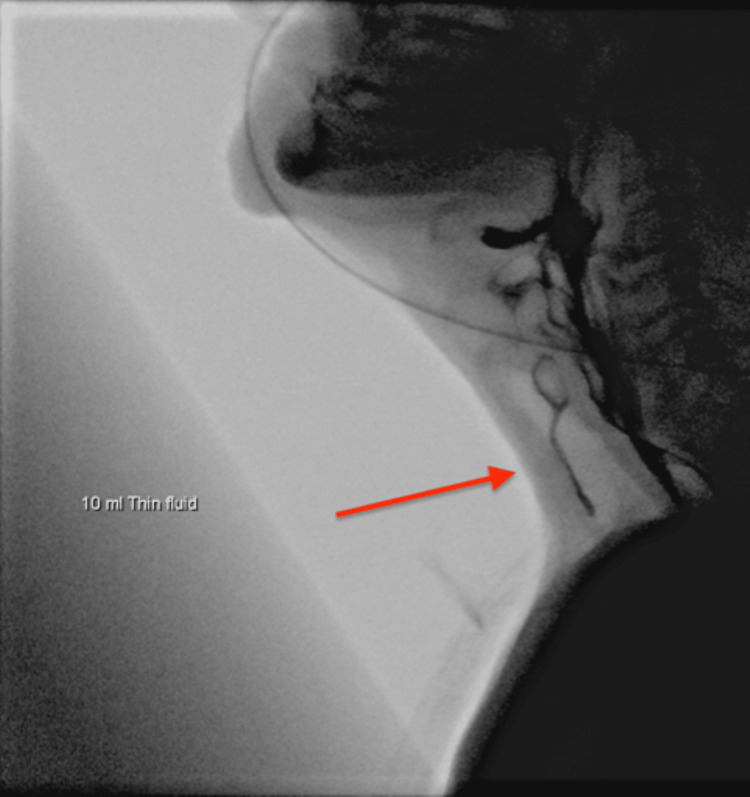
VFSS demonstrating silent aspiration (arrow) and external compression of the oesophagus by a large anterior osteophyte Original X-ray image from Royal Hobart Hospital, Australia, 2025, reproduced with permission from Royal Hobart Hospital Radiology Department VFSS: Videofluoroscopic swallow study
